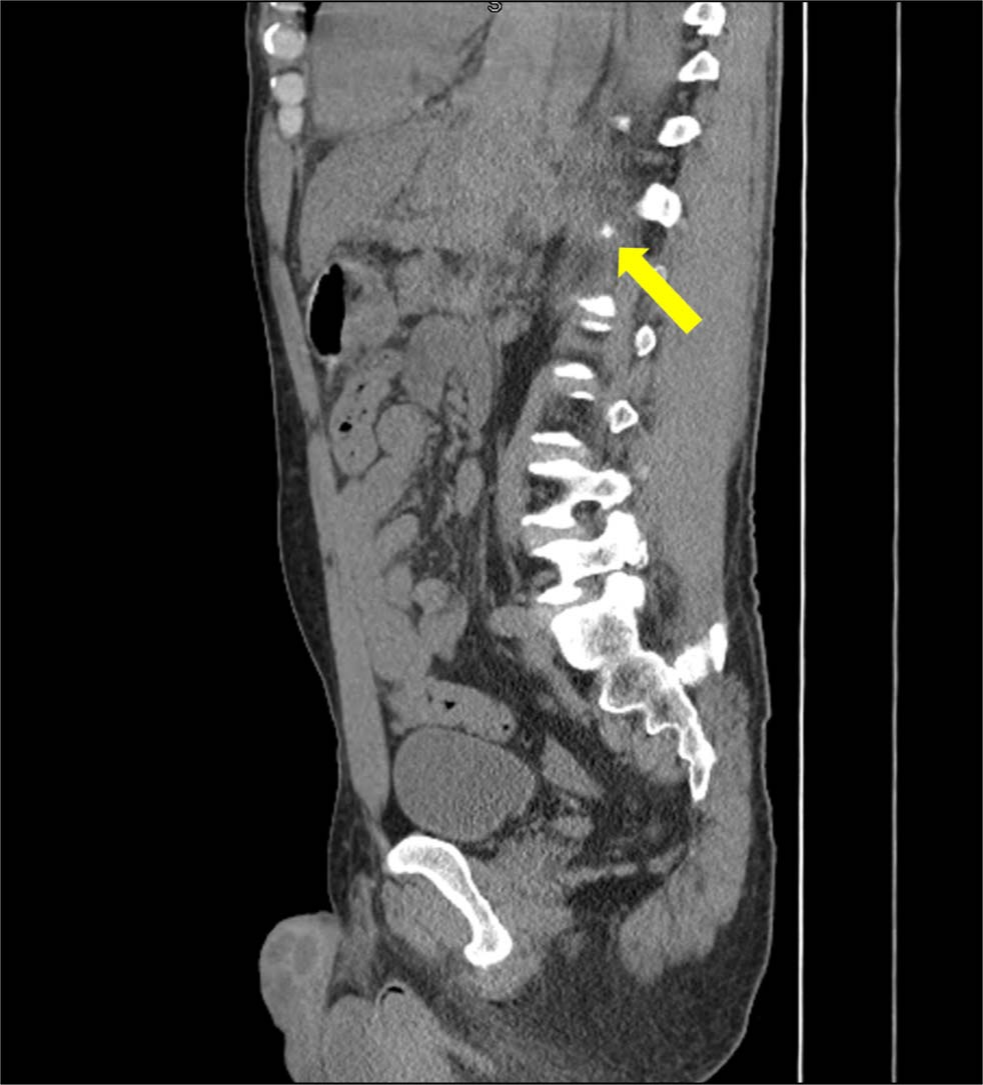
Noncontrast computed tomography chest abdomen and pelvis sagittal view showed relatively higher density left pleural effusion (yellow arrow) and deep pelvic fluid.
